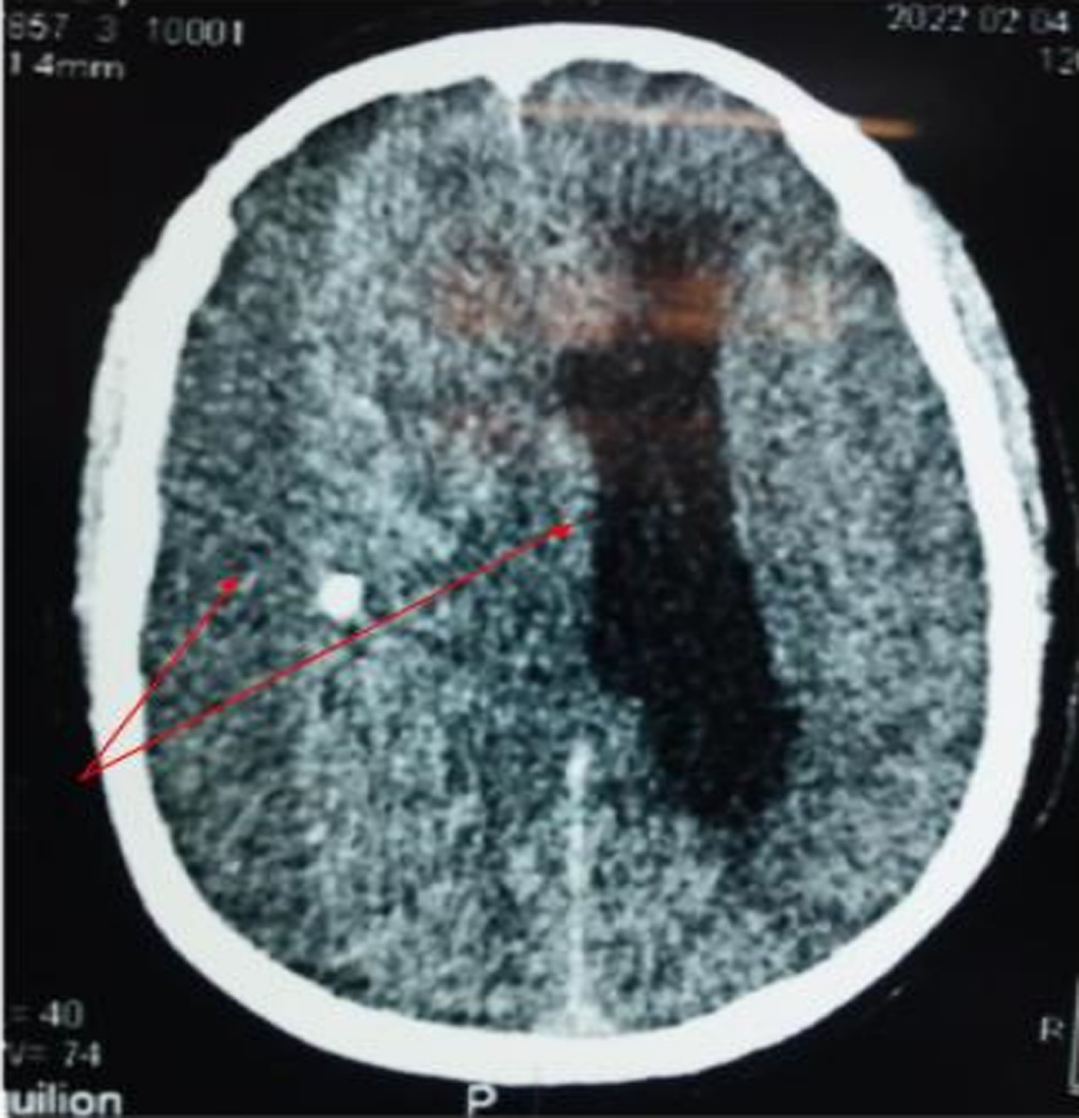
Cerebral CT image, an axial section showing a voluminous right hemispheric chronic subdural hematoma with mass effect.
